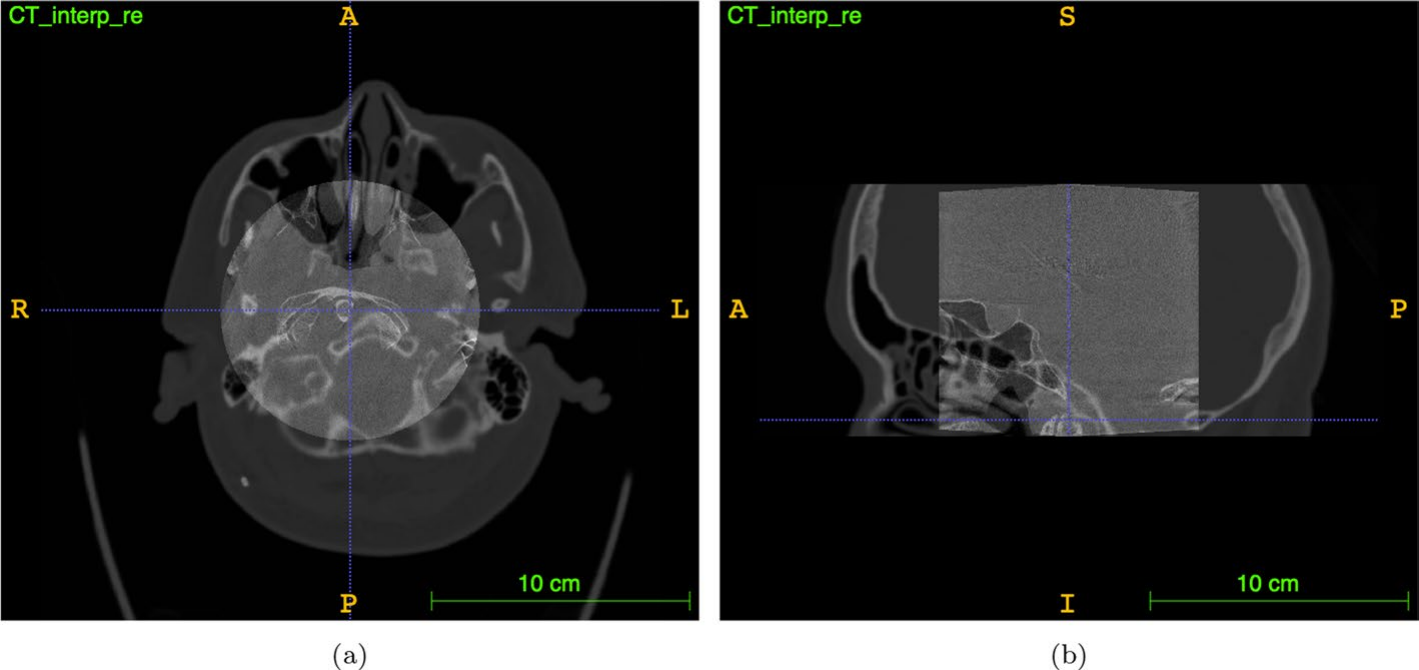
Transparent overlay of unregistered data: a image shows axial slice and b image shows mid-sagittal slice of DCTm and MS-CT in one patient (after interpolation, CT is cut along z for computational purposes)
